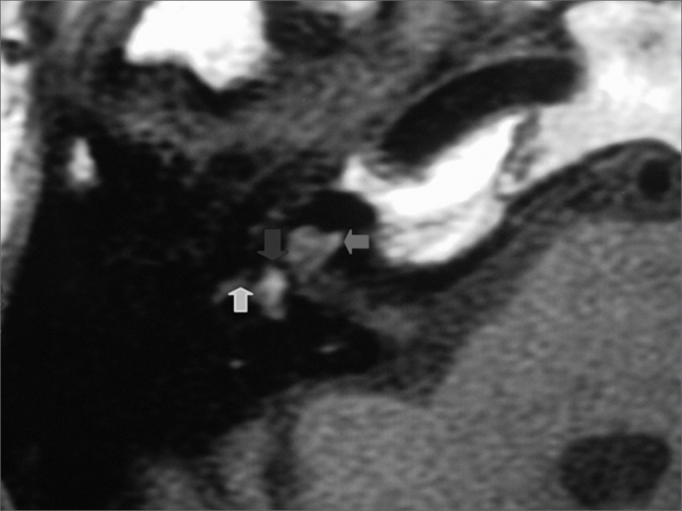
Magnetic resonance imaging of the temporal bones; axial slice, T1-weighted image with no endovenous contrast, showing a hyperintense signal in the cochlea (red arrow), the vestibule (blue arrow) and the anterior portion of the lateral semicircular canal (green arrow).
